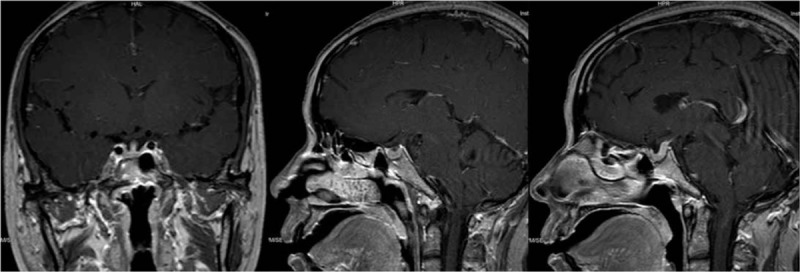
Enhanced MRI of sellar region in 2009. MRI before radiosurgery showed T1 low signal nodular intensity (0.5 × 0.9 × 0.4 cm) with homogeneous nodular enhancement of posterior pituitary, and also presented an enlarged pituitary stalk.
